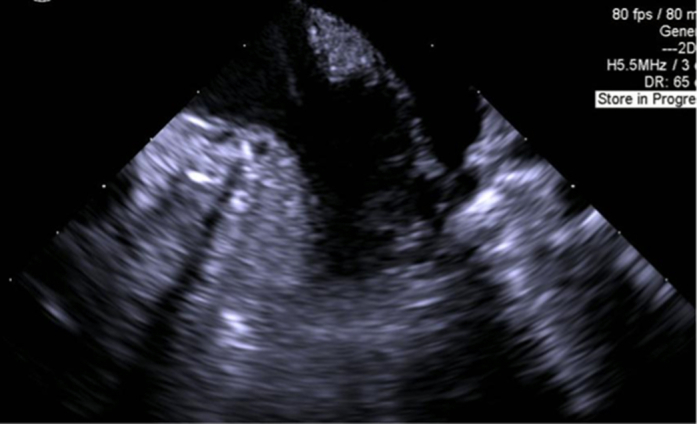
Post-Thrombectomy Transesophageal Echocardiogram Absence of thrombus confirmed in all cases.
